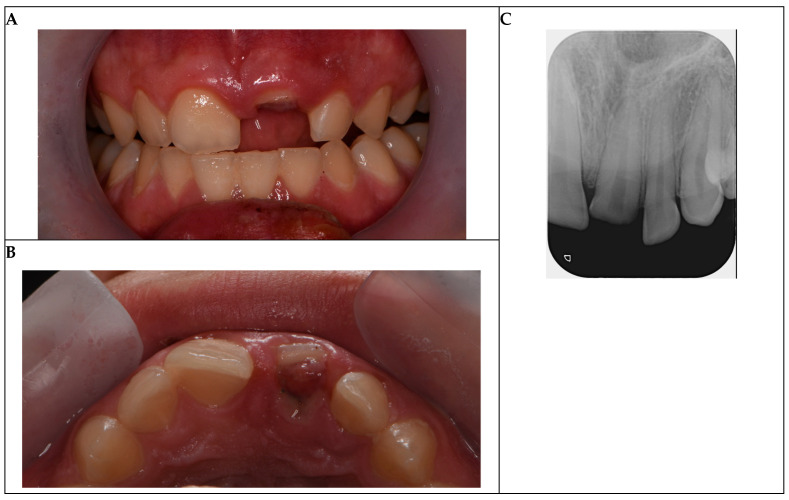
Initial situation. (A) Buccal view of the complicated crown fracture of tooth 21, showing subgingival extension and pulp exposure. (B) Occlusal view of the fractured tooth. (C) Pre-operative periapical radiograph confirming intact periodontal ligament space, mature root apex, and no periapical pathology.
